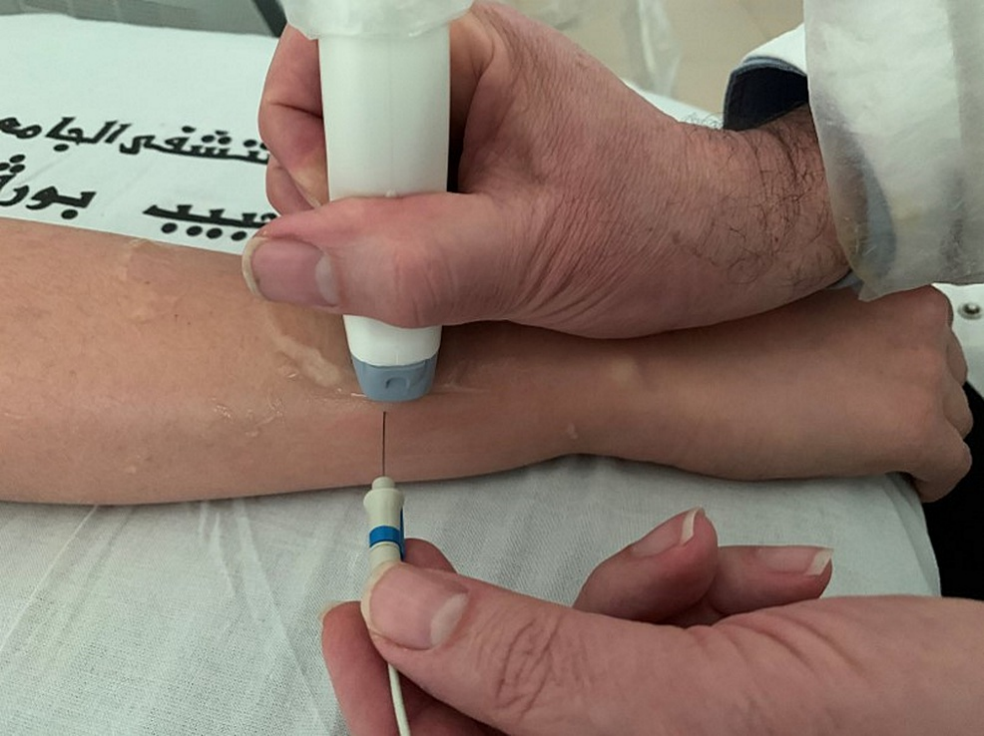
Performing the ultrasound of the forearm The ultrasound probe is placed at the level of the distal part of the posteromedial side of the forearm, i.e., 4 to 5 cm above the wrist. The electromyography (EMG) needle is then inserted laterally and perpendicular to the medial edge of the forearm. Note: This individual depicted in this figure was a resident who provided consent for demonstration purposes.
